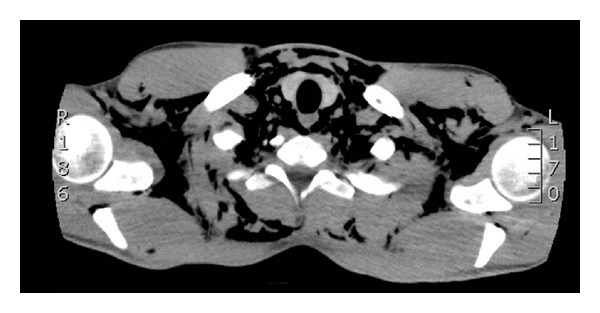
Axial CT image showing gross subcutaneous emphysema.
